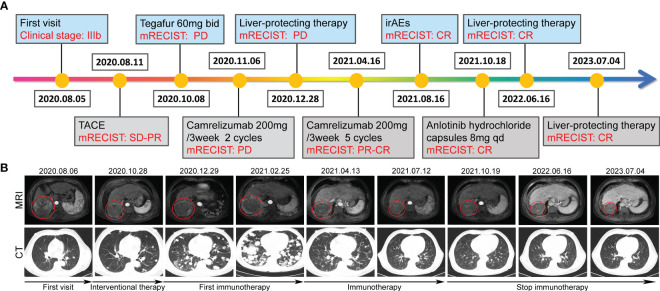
Tumor responses after therapy. (A) Timeline of disease status and corresponding treatment regimens. (B) Time line of treatments of the patient and changes in MRI and CT of the liver and lung during treatments, the red circle location of the tumors.
